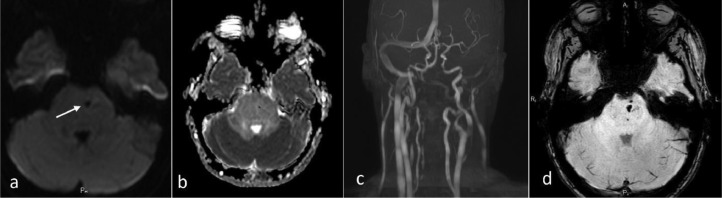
Additional representative MRI data before treatment. DWI (a), ADC-map (b), time-of-flight angiography (c) and SWI data (d) that resulted in a consensus diagnosis of “probable CLIPPERS.” Features of CLIPPERS pathophysiology: there was no restricted diffusion such as an insult or tumor, no appreciable vascular stenosis or malformations (we noted unremarkable asymmetry of vertebral arteries); deep curve vessel fragments (prominent veins) were seen on DWI and SWI (arrows).
